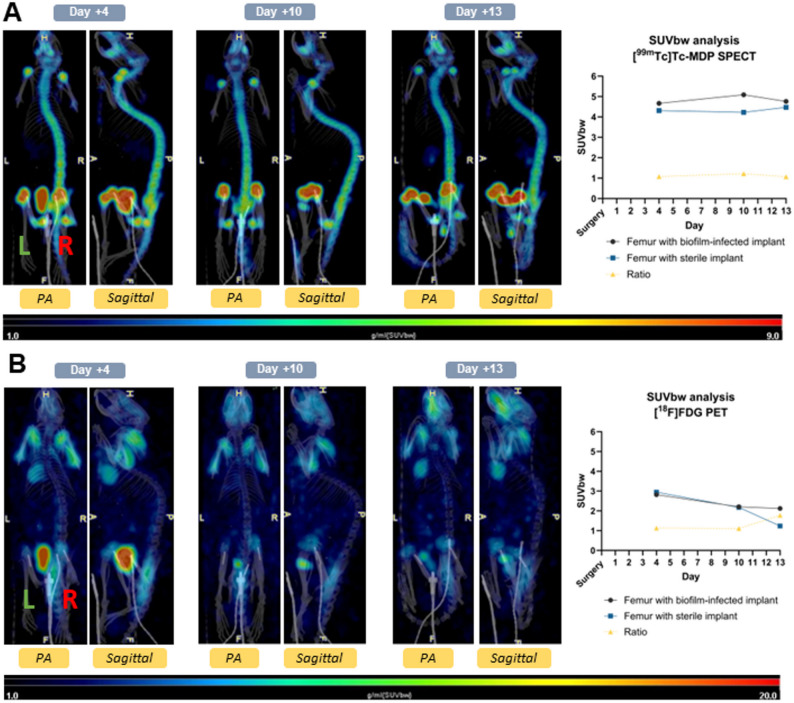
SPECT/CT scans using [^99m^Tc]Tc-MDP (bone scintigraphy) and PET/CT scans with [^18^F]FDG demonstrate comparable uptake at both biofilm-infected and sterile implant sides consistently throughout the study period.Legend: The green L indicates the left sterile implant and the red R indicates the right biofilm-infected implant. The SUVbw and ratio of the femurs with implants are displayed. Posteroanterior (PA) and sagittal planes are depicted. (a) [^99m^Tc]Tc-MDP SPECT shows uptake in both implant sites. The ratio SUVbw between femur with biofilm-infected implant and femur with sterile implant on days 4, 10, and 13 post-surgery was 1.07, 1.22, and 1.07, respectively. (b) [^18^F]FDG PET shows uptake in both implant sites. The ratio SUVbw between femur with biofilm-infected implant and femur with sterile implant on days 4, 10, and 13 post-surgery was 1.13, 1.10 and, 1.77, respectively
